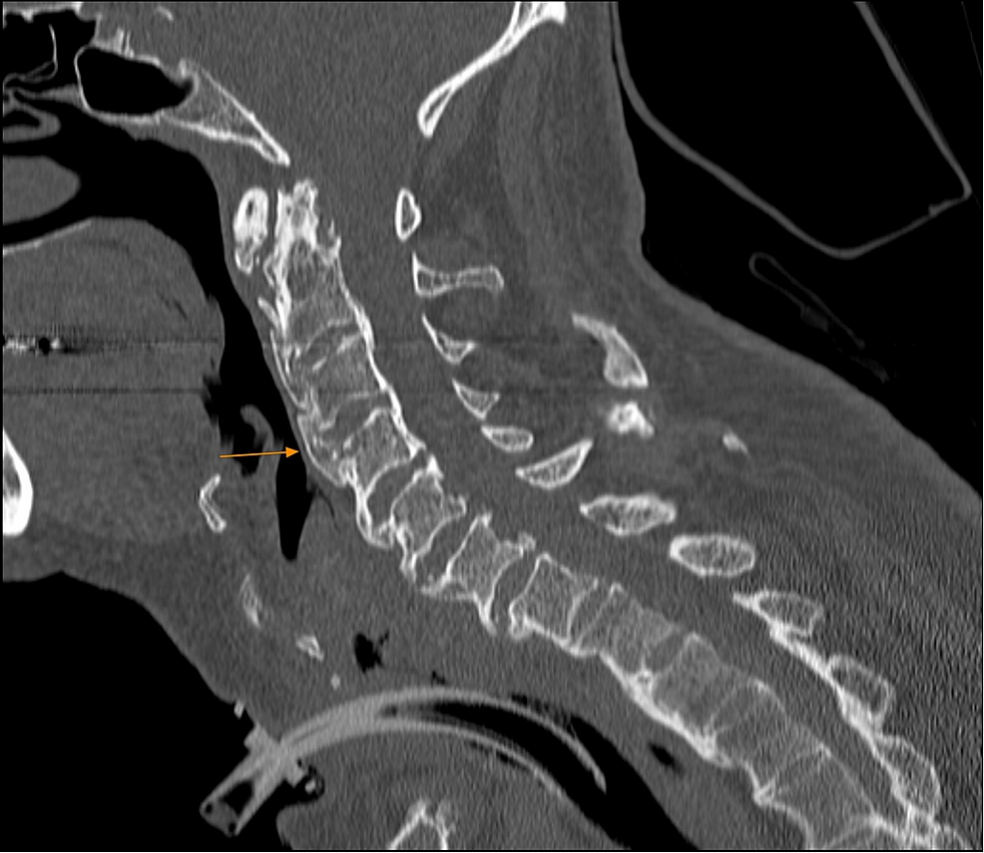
Preoperative sagittal cervical computed tomography demonstrating bridging of the vertebral bodies. The arrow pointing to the bridging of the anterior wall of the C3-C4 vertebral level.
